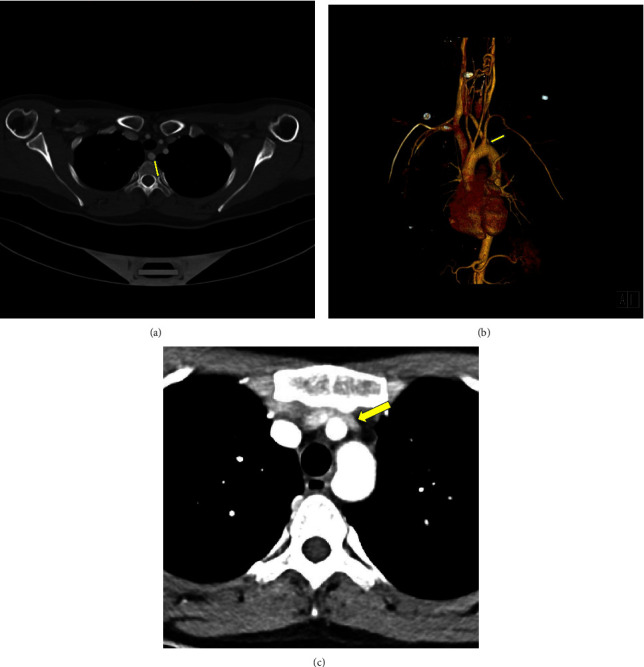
(a) Axial contrast-enhanced CT images (CTA neck) demonstrate the anatomical variation of an aberrant right subclavian artery (yellow arrow) with its course posterior to the esophagus. (b) Volume rendered 3D reconstruction demonstrates an aberrant right subclavian artery (arrow) arising distal to the origin of the left subclavian artery and traverses the mediastinum posterior to the esophagus. (c) Axial contrast-enhanced CT images (CTA chest) examination performed in the inspiratory phase demonstrates mild compression of the brachiocephalic vein (yellow arrow), however, patency is maintained. Anteroposterior (AP) diameter of 7.4 cm on inspiratory phase.
